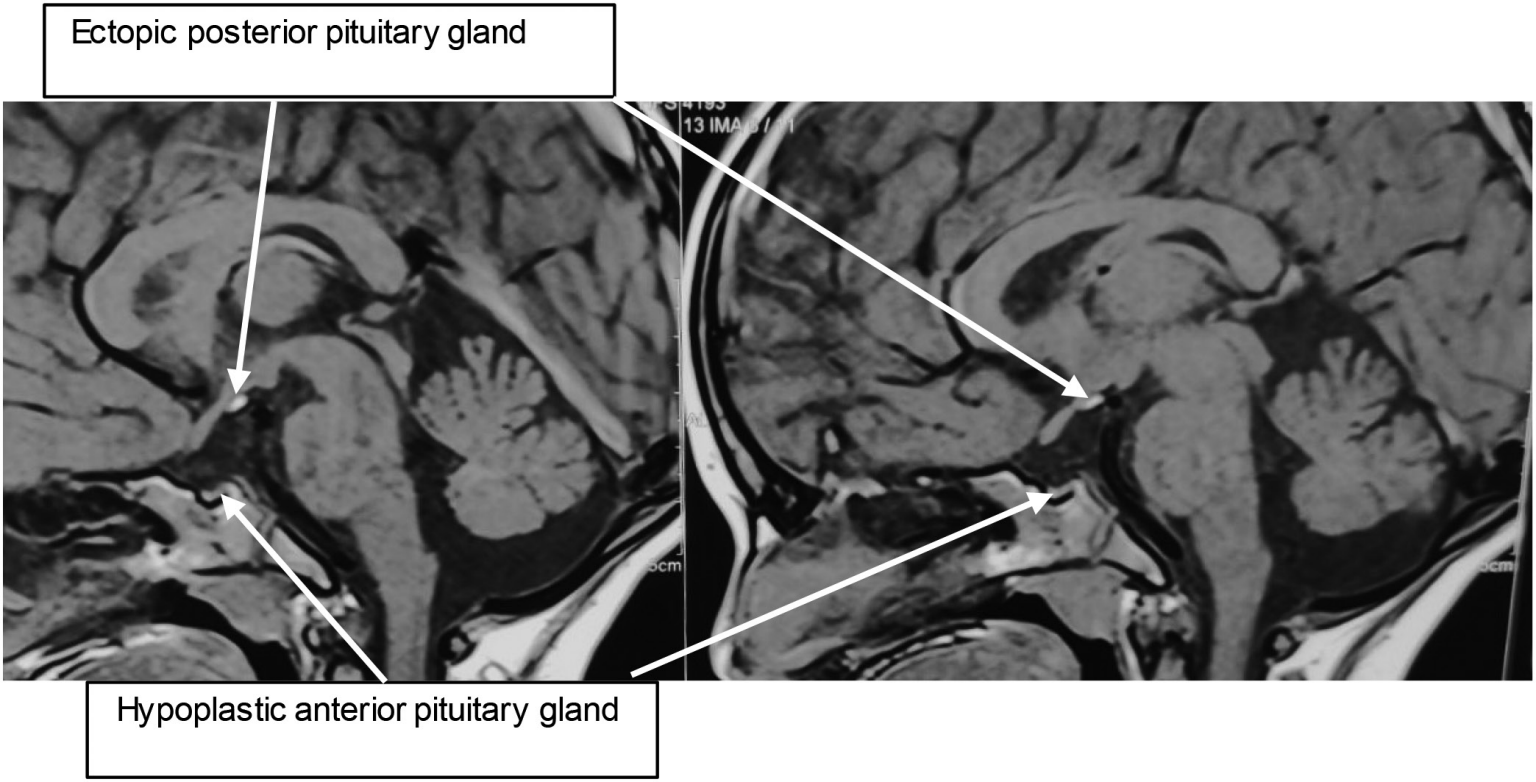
Sagittal T1 weighted MRI with the classic triad—pituitary hypoplasia, absent stalk and ectopic posterior pituitary. The pituitary gland is relatively small in size and measures 4.1 × 3.6 × 1.08 mm (TR × AP × CC). No definite nodule could be identified in plain or dynamic scans. Tl bright posterior pituitary gland is not visualized in the pituitary fossa but is seen posterior to the base of the infundibulum and inferior to hypothalamus.
